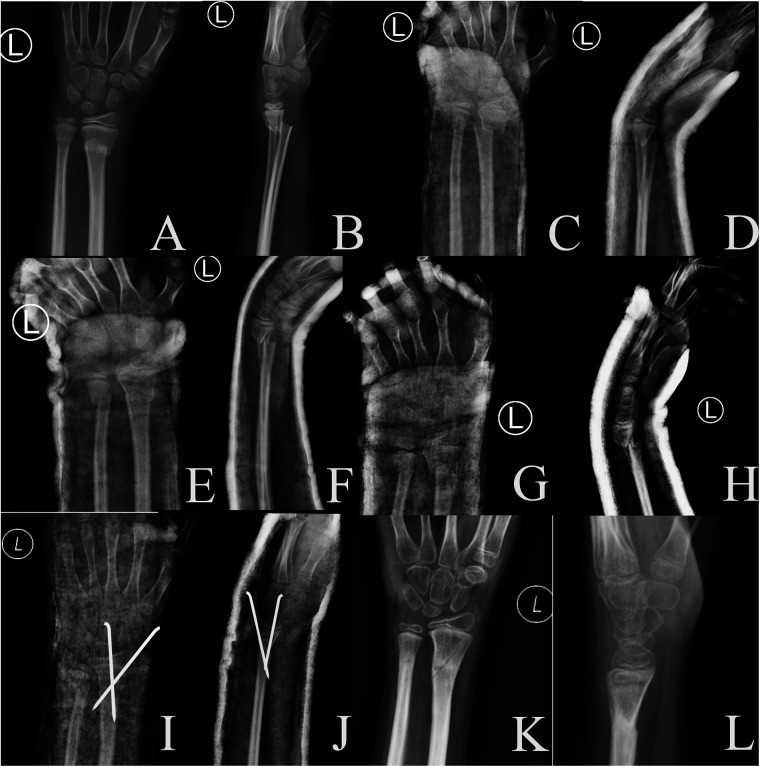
X-rays of an 11-year-old male boy who suffered a fracture of the distal end of the left radius and ulna due to a fall. (A,B) preoperative anteroposterior and lateral radiographs; (C,D) first reduction anteroposterior and lateral radiographs; (E,F) second reduction anteroposterior and lateral radiographs; (G,H) third reduction anteroposterior and lateral radiographs; (I,J) post-operative reduction with prying lever technique Anteroposterior and lateral radiographs; (K,L) Anteroposterior and lateral radiographs after removal of K-wires after healing.
